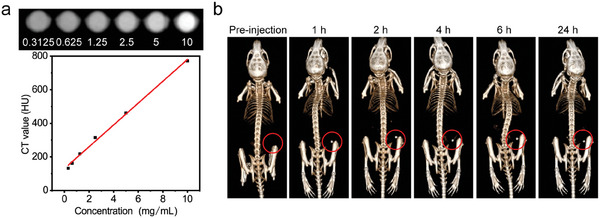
CT imaging of Ir@WO_3−x_ nanoreactors. a) Curve of Ir@WO_3−x_ nanoreactors solution concentrations versus CT values. From left to right: 0.3125; 0.625; 1.25; 2.5; 5; 10 mg mL^−1^. b) CT images were acquired at different timepoints after the intratumoral injection of Ir@WO_3−x_ nanoreactor solution (5 mg·kg^−1^). Significant enhancement of CT contrast is visible within the red dashed circle.
